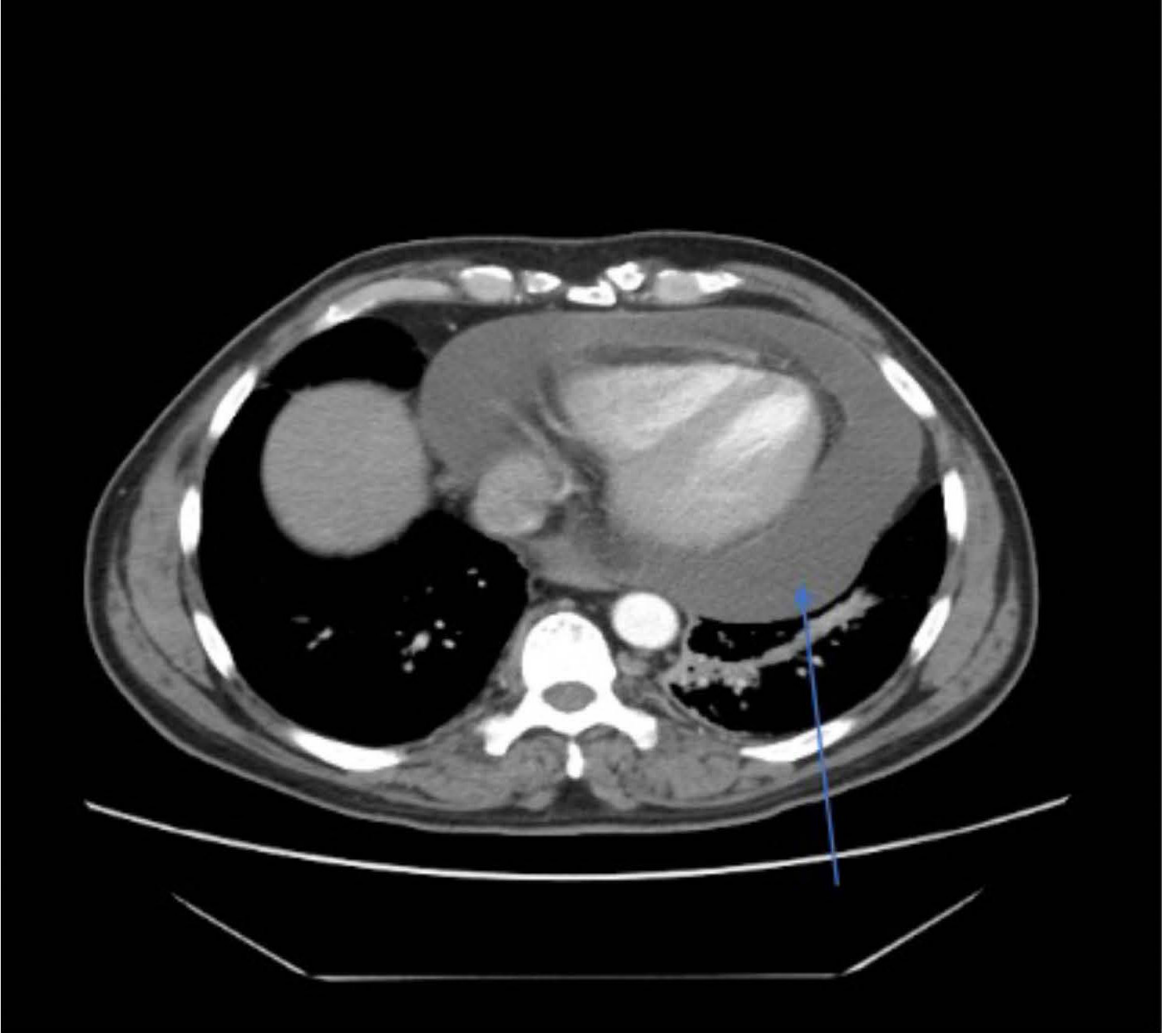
Axial plane: CT scan of chest showing large pericardial effusion (blue arrow).
